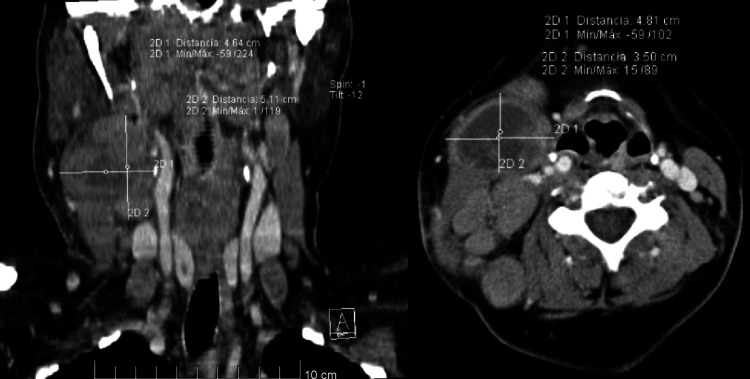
CT scan of the neck showed a mass with no compression of nervous or vascular structures.
